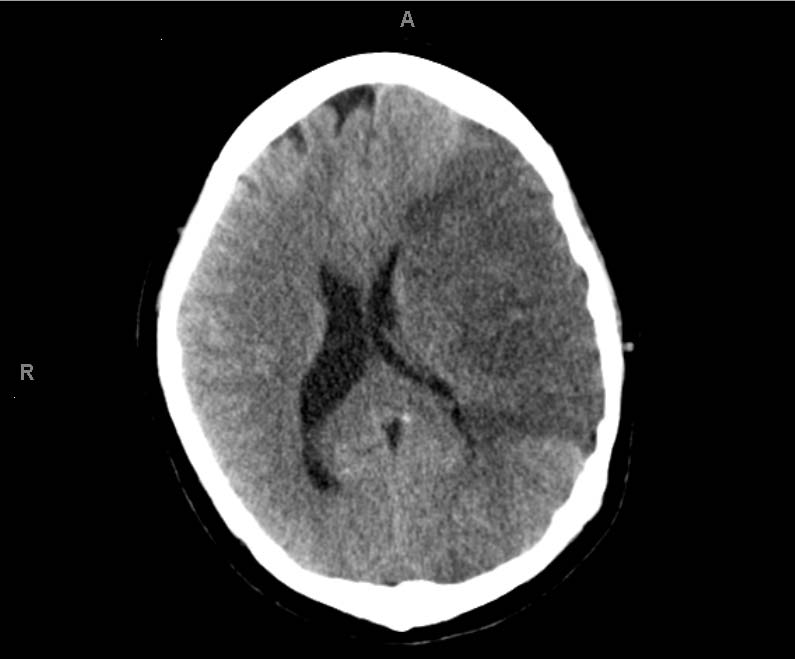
CT scan cerebrum performed on the last day of the patient's life.
